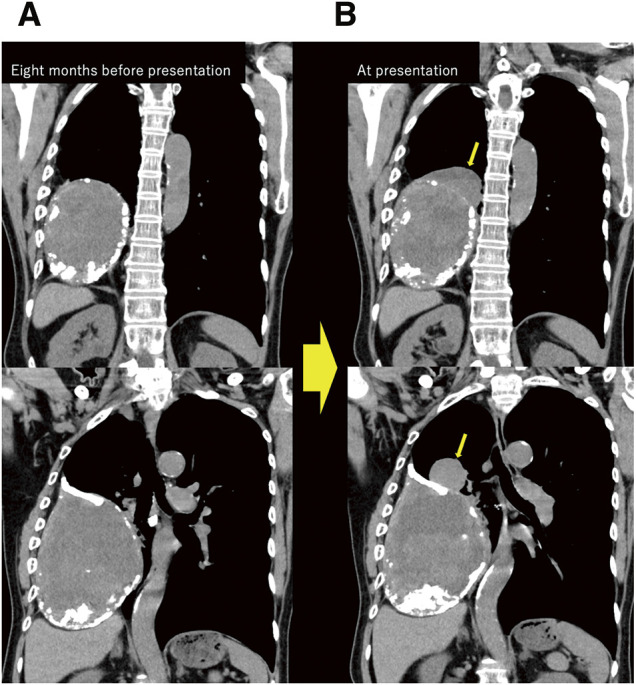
Comparison of coronal contrast-enhanced chest CT images in the equilibrium phase obtained 8 months before presentation (A) and at presentation (B), showing a mass in the right pleural cavity with peripheral calcification and enhancement of the lesion wall. 2 cranially projecting components developed during the interval (arrows).
